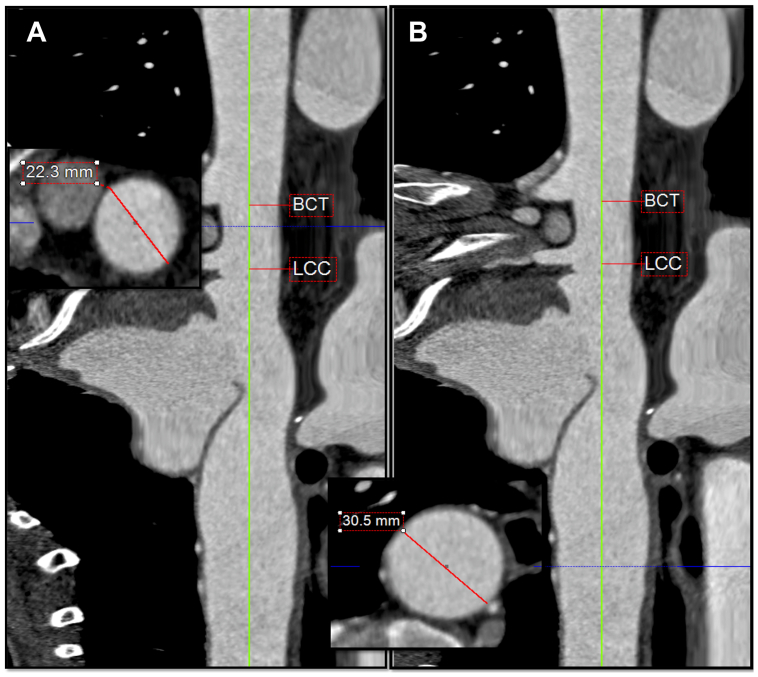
Multiplanar computed tomography angiography (CTA) reconstructions demonstrating the discrepancy between the proximal (A) and distal (B) aortic diameters at the intended landing zones. The proximal landing zone measured 22 mm, whereas the distal thoracic aorta measured approximately 30 mm. This diameter mismatch necessitated the implantation of two overlapping thoracic stent grafts of different diameters to ensure optimal apposition and adequate proximal and distal sealing. *BCT*, Brachiocephalic trunk; *LCC*, left common carotid.
